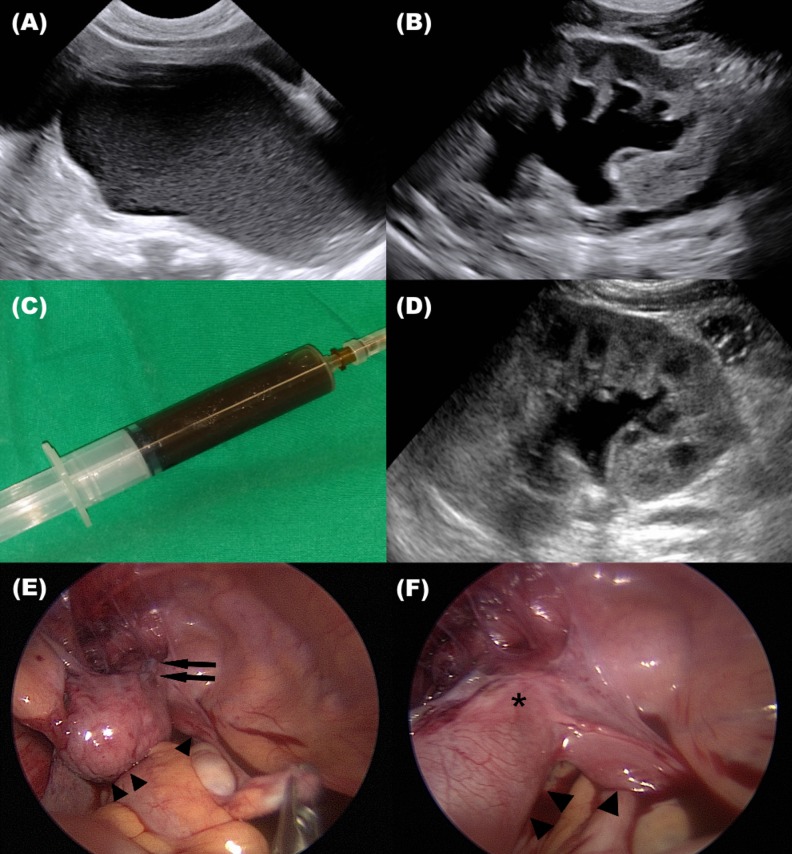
Representative images of two cases that underwent operation. (A) Ultrasonography of a two-month-old girl with fluid-filled vaginal distention. (B) Contralateral hydronephroureterosis due to pressure from the vaginal mass. (C) Aspirated hemorrhagic vaginal fluid. (D) Resolution of hydronephroureterosis after vaginal fluid aspiration. (E, F) Laparoscopic features of a four-year-old girl with obstructed hemivagina and ipsilateral ureter insertion to hemivagina. * Arrow head: bilateral uterus, Arrow: ipsilateral ureteral stump, Asterisk: ipsilateral obstructed hemivagina.
